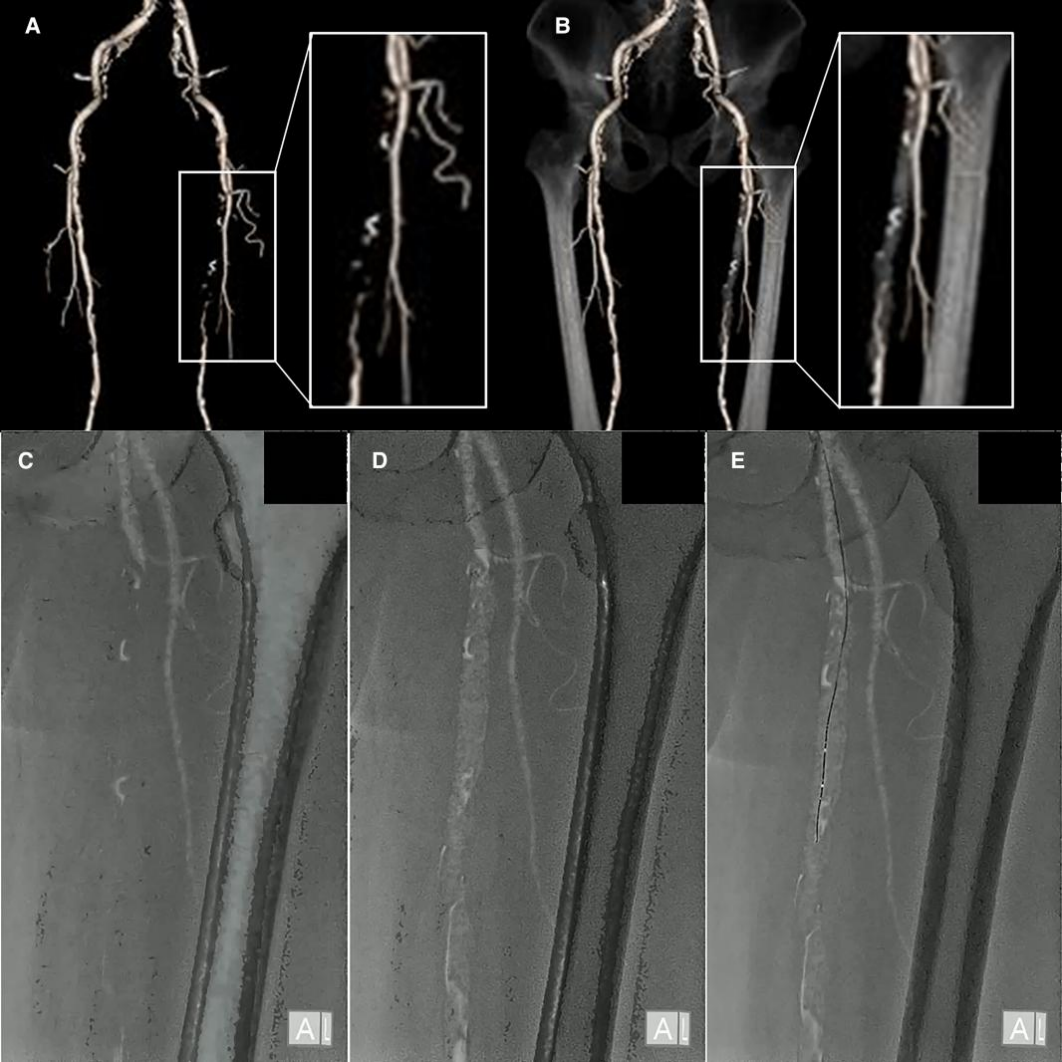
Contrast-enhanced computed tomography and fluoroscopy.
